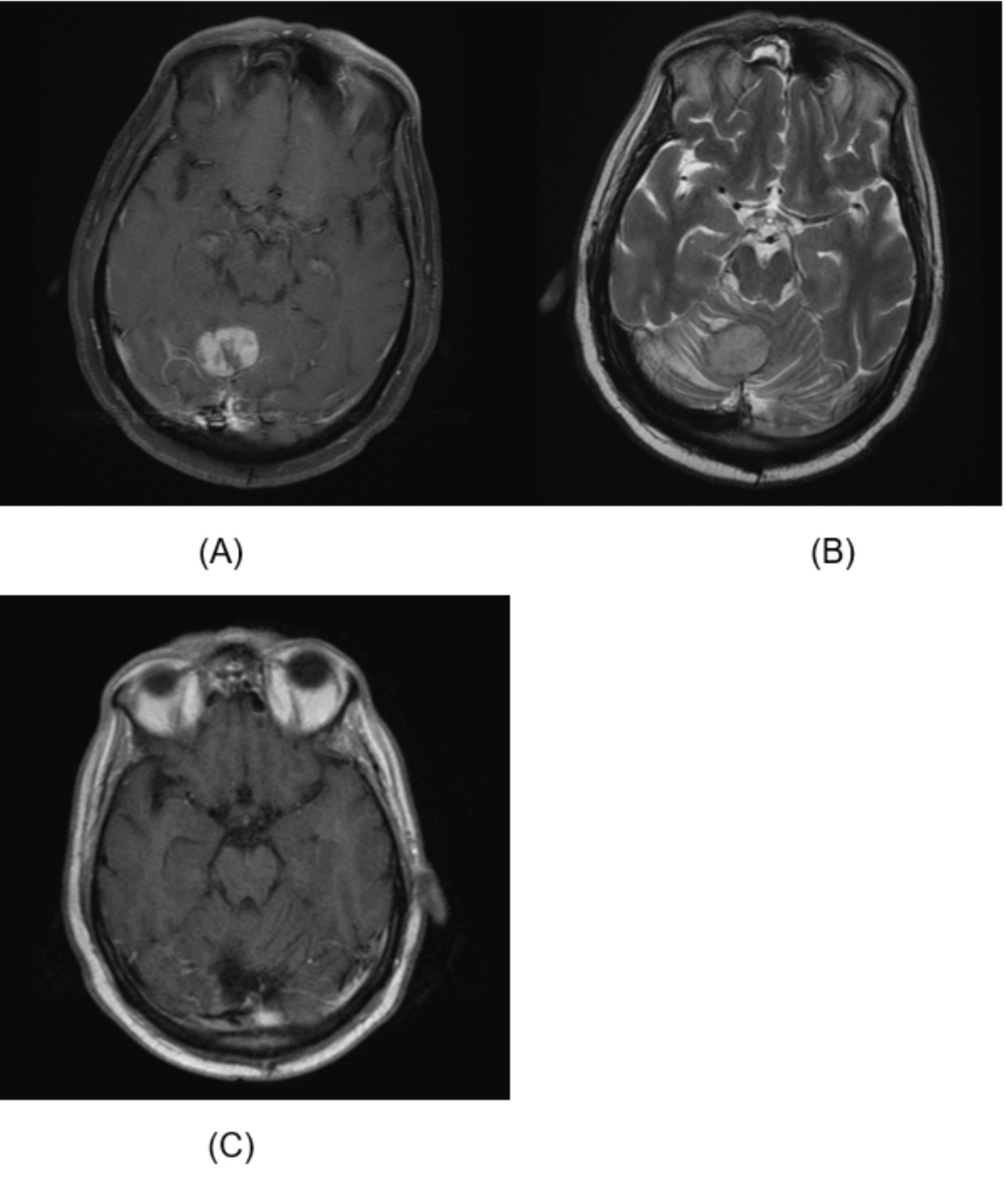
MRI of cerebellar mass (A) Axial T1 post-contrast image of the brain demonstrates an avidly enhancing cerebellar mass arising from the vermis. (B) Axial T2 MR image shows surrounding vasogenic edema and effacement of cerebellar sulci. (C) Post-surgical axial T1 post-contrast image of the brain demonstrates a surgical cavity with no evidence of residual neoplasm.
